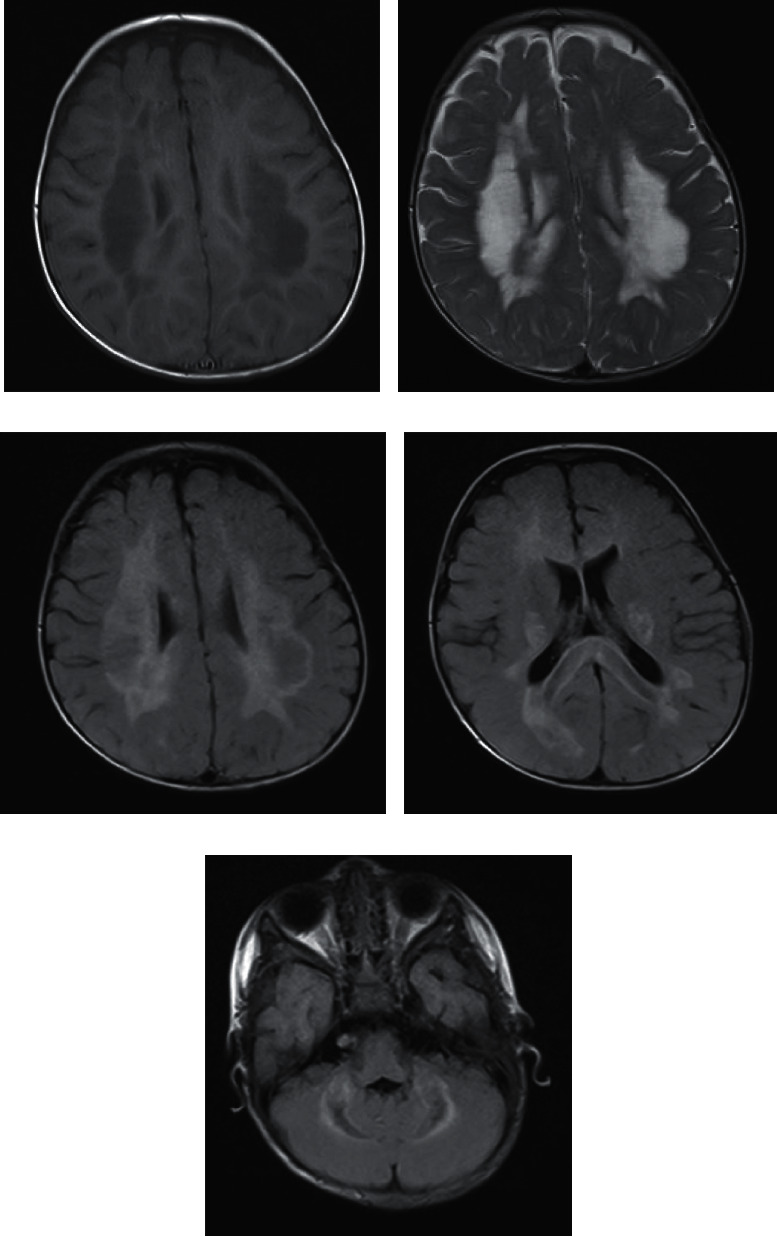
Axial brain MRI of a 1-year-old ADEM patient, who presented with stiffness of bilateral upper and lower extremities, with history of recent upper respiratory tract infection, showing large, bilateral, diffuse lesions, predominantly affecting the deep white matter, the corpus callosum, and bilateral cerebellar peduncles. The lesions are hypointense on unenhanced T1WI (a), hyperintense on T2WI (b), and partially suppressed on FLAIR (c–e).
